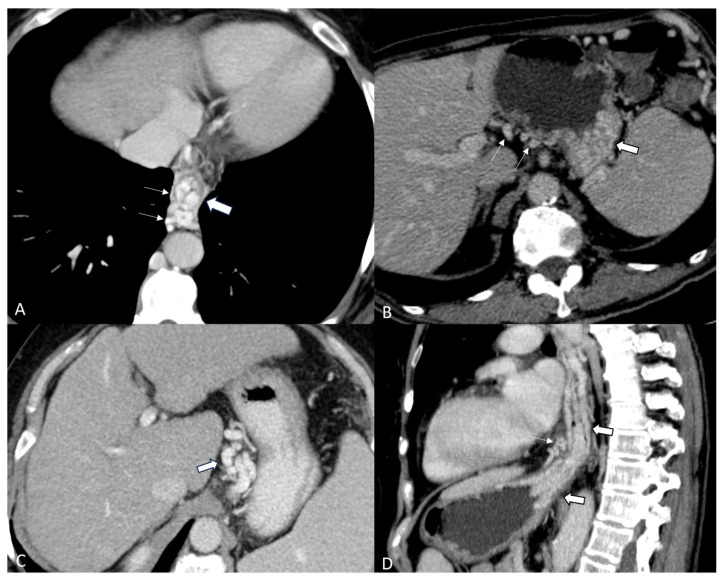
(A) CT appearances of esophageal varices show as intraluminal protrusion with scalloped margins (large arrow). Paraoesophageal varices (thin arrows) appear as well-defined, round, tubular, or serpentine structures situated outside the wall of the esophagus in the mediastinum. (B) Gastric varices (large arrow) located submucosal in the postero-superior aspect of gastric fundus and presence of dilated veins on the lesser curvature of stomach (thin arrows). (C) Left coronary vein varices (large arrow). (D) Gastroesophageal varices extend from inferior esophagus to the upper pole of the stomach (large arrows), in association with paraoesophageal varices (thin arrow).
